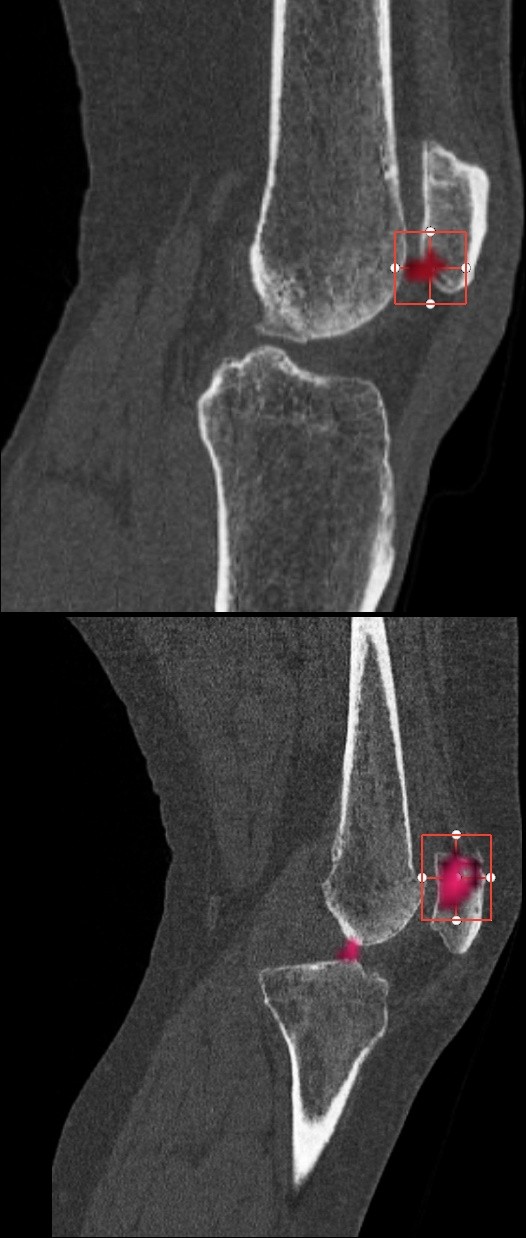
SPECT uptake region analysis. Using a higher threshold (four times the mean of the reference region) showed a higher value for patient 2's uptake region (bottom figure, upper patellar pole) as compared to patient 1, (top figure, lower patellar pole).
